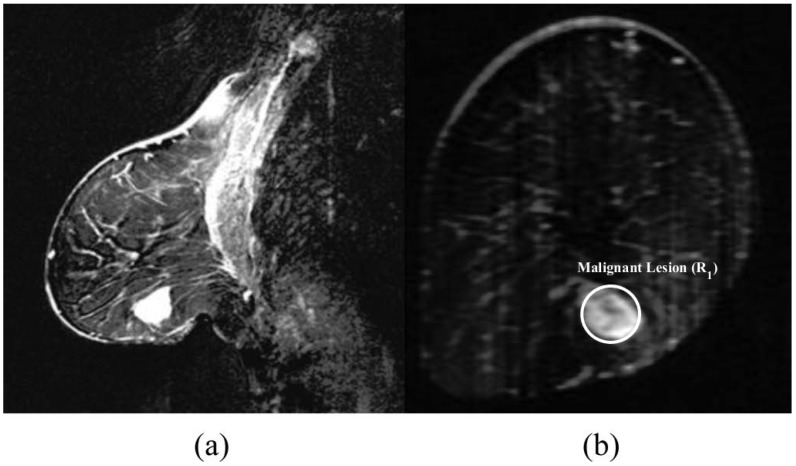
MRI scan of Patient 1 (from [24]): (a) saggital view; and (b) coronal view. For Figure 2, IEEE License received. License number 4274890619942.
